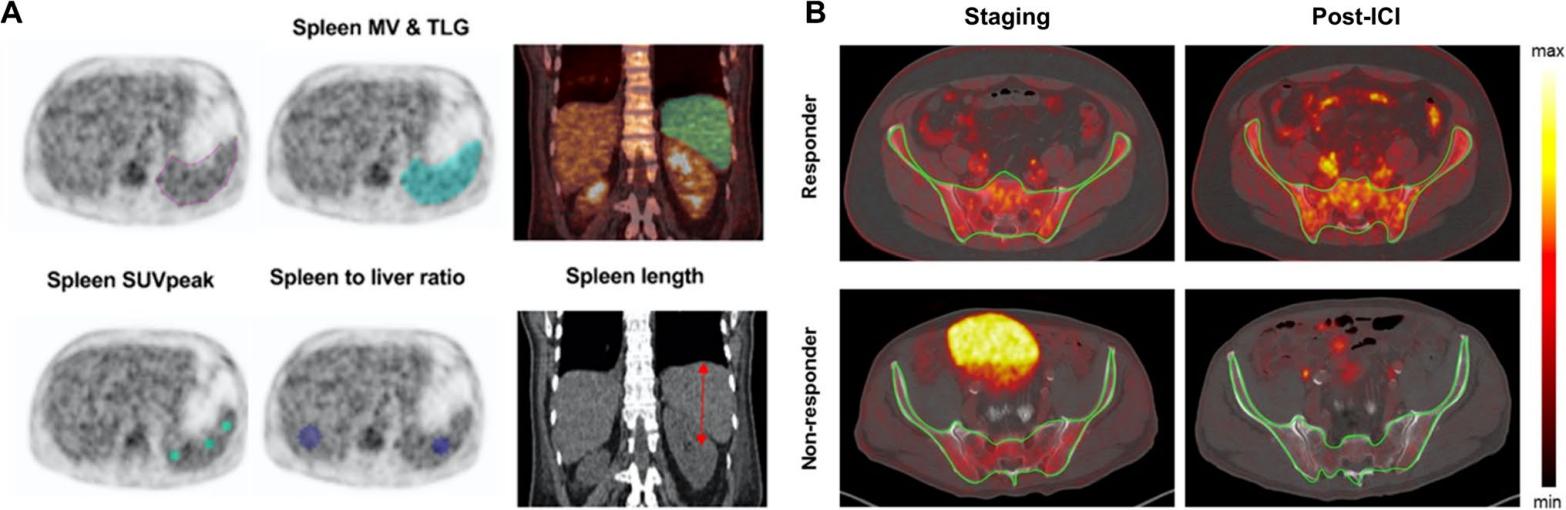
^18^F-FDG PET imaging can be used to monitor metabolic patterns in lymphoid organs for the evaluation of systemic immune response after ICI therapy. A Methodology for measurement of uptake in the spleen to assess ICI outcome. B Representative PET/CT images showing the baseline scan and the follow-up scan of BM after the initiation of ICI treatment. The significantly enhanced ^18^F-FDG uptake within the bone was a consequence of successful treatment. (The researches were originally published in Eur J Nucl Med Mol Imaging. 2021 Jul;48(8):2573-2585 [52] and Theranostics. 2020 Jan 1;10(2):925-937 [53], respectively)
